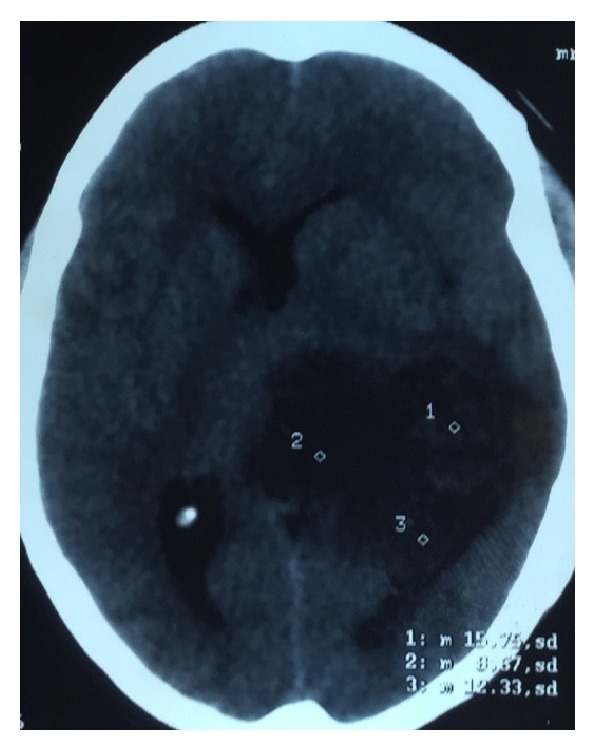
Recurrence of lesion on CT-scan after surgery.
